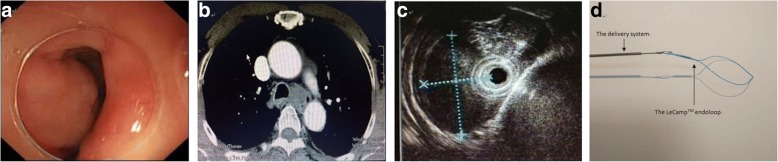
Evaluation of the SMT and the LeCamp™ endoloop device. a an endoscopic image revealed a protruding submucous lesion with a smooth surface. b CT image showing a 1.8 cm × 5.2 cm × 2.9 cm soft tissue mass. c EUS image showing a hypoechoic mass originated from the muscularis propria layer. d the LeCamp™ endoloop and its delivery system
